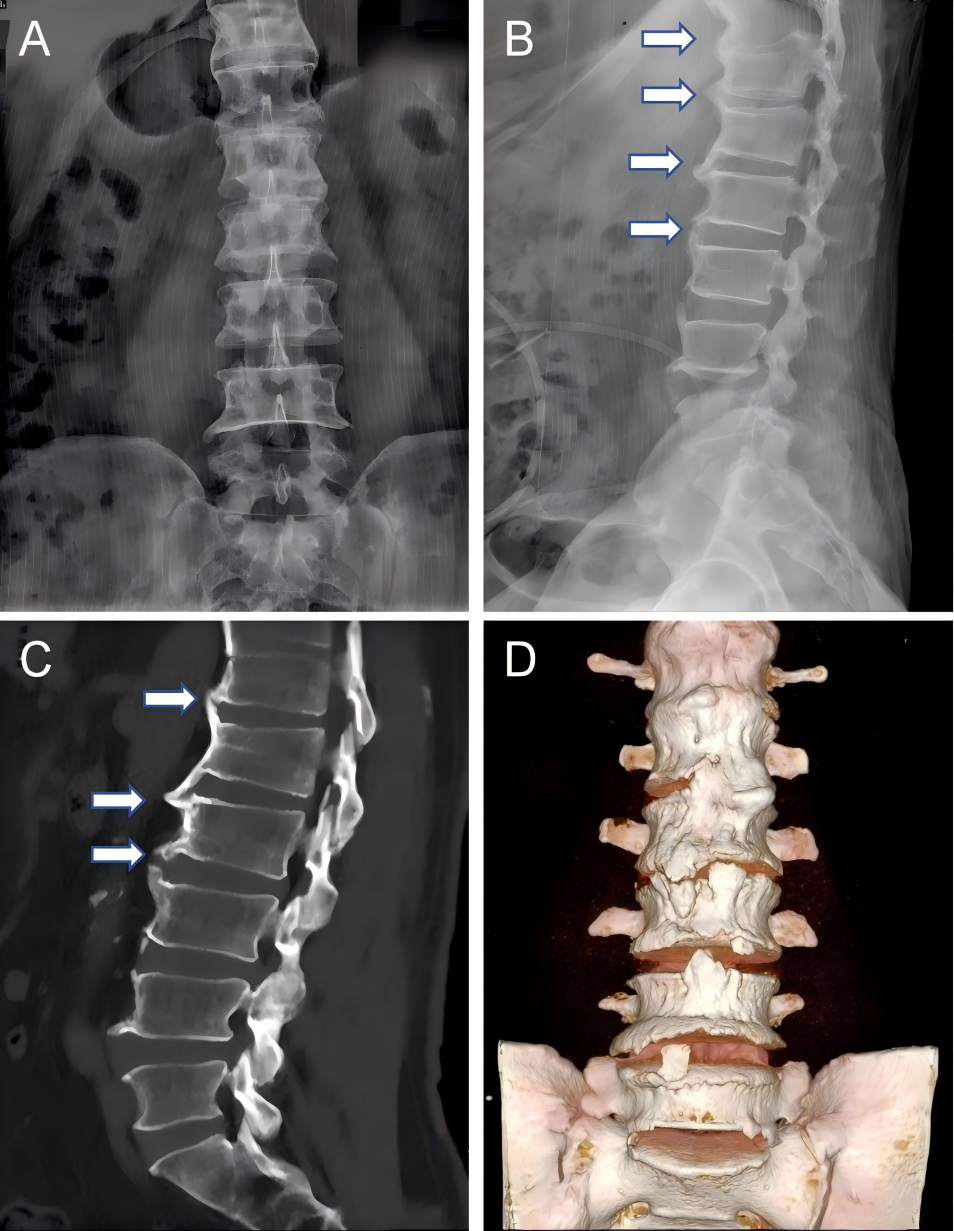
Representative imaging features of DISH in an elderly male patient. Flowing, bridging ossification is present anterior to the vertebral bodies (arrows). The sacroiliac joints show no significant erosion, sclerosis, or bony ankylosis. (A) Anteroposterior radiograph of the lumbar spine. (B) Lateral radiograph. (C) Sagittal CT reconstruction. (D) Three-dimensional CT reconstruction.
